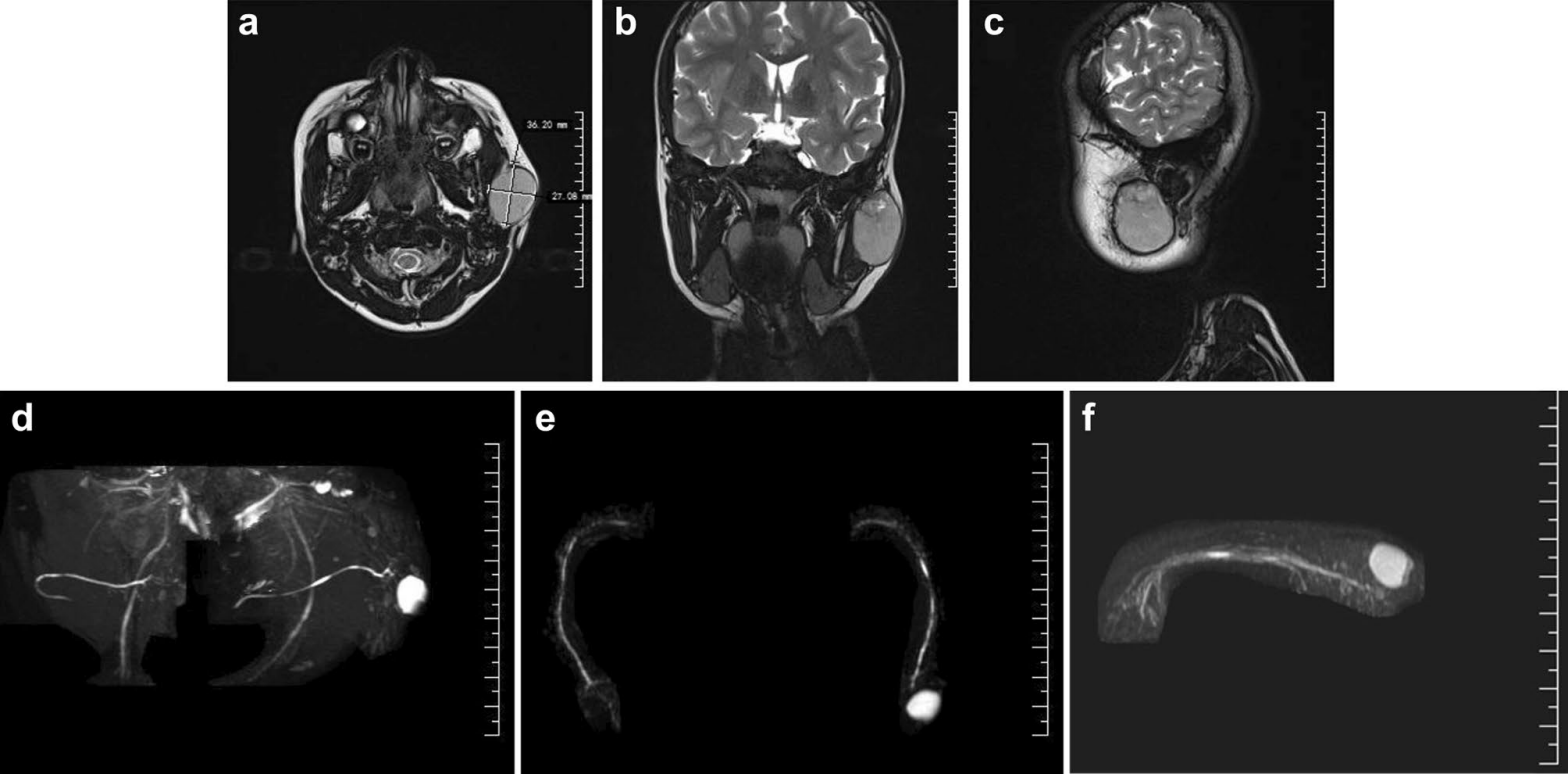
Magnetic resonance imaging (MRI) imaging findings of typical SCSG. On MRI, tumors tend to be roundish in shape, with clear boundaries. T2 images showed long signals or mixed long and short signals; (a) horizontal plane; (b) coronal plane (c) sagittal plane. MRH detected lesions located in the posterior lower pole of the parotid gland; (d) coronal plane (e) horizontal plane (f) sagittal plane.
